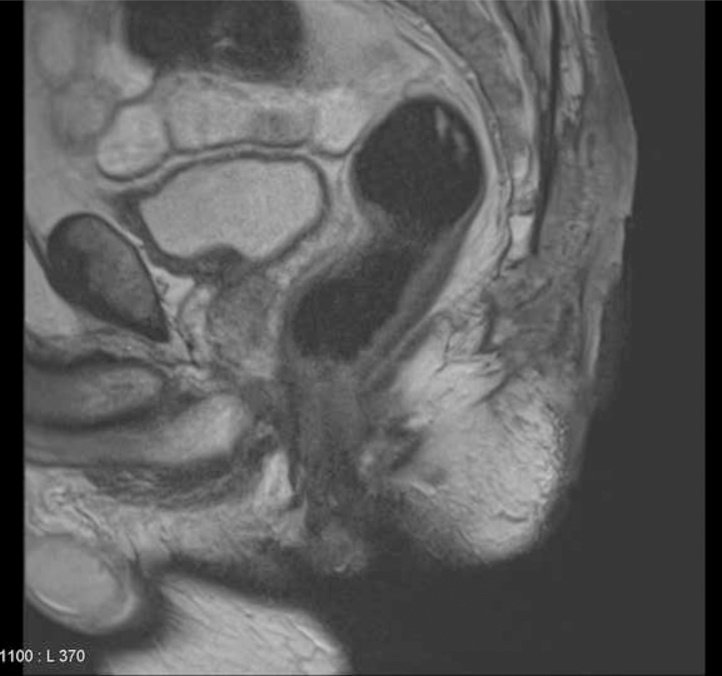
MRI imaging before neoadjuvant treatment (sagital view).
